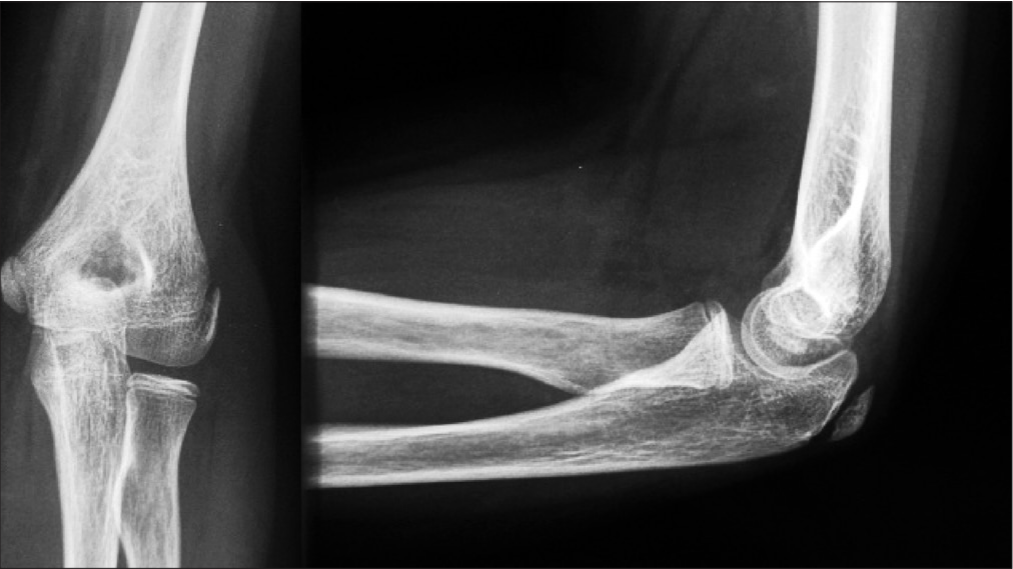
Initial X-rays of the left elbow showing only a dense lateral condyle
